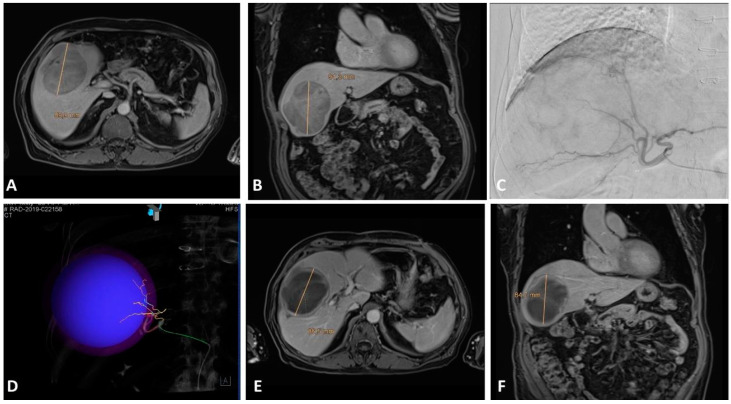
(A,B) Axial and coronal contrast-enhanced MRI images showing a 9 cm dishomogenously hypointense lesion in the right hepatic lobe in a BCLC-B patient. (C) Pre-treatment diagnostic angiography showing tumor-feeding arteries. (D) CBCT coronal VR reconstruction showing target volume and color-coded tumor-feeding arteries. (E,F) Axial and coronal contrast-enhanced MRI images showing 3-month post-DEB-TACE results with great tumor necrosis.
